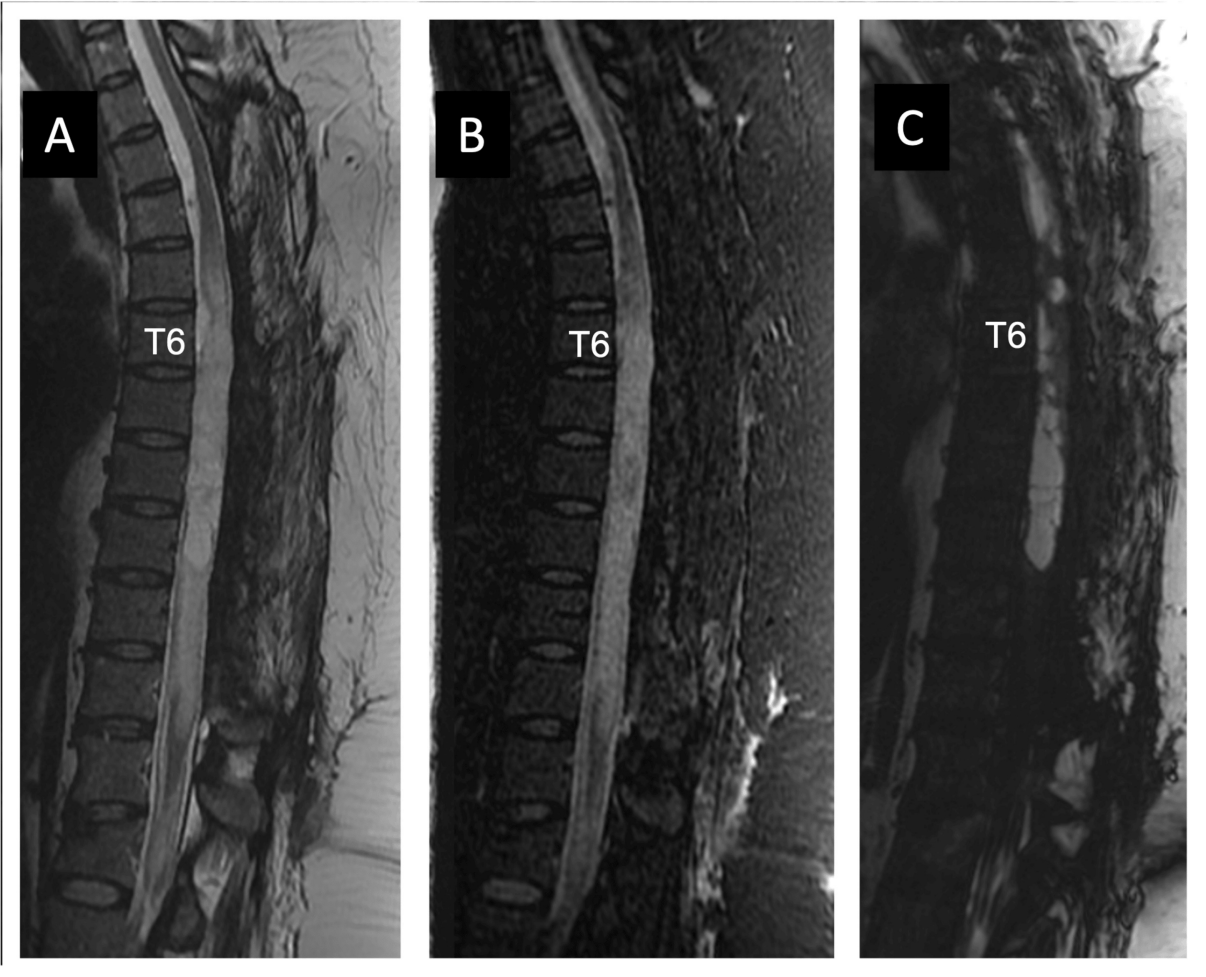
Sagittal T2 (A), T2-STIR (B), and T2-3D (C) MRI, four months after the extended laminectomy from T7 to T11 levels, shows signs of arachnoiditis with adhesions, septations within the dural sac, distortion and displacement of the thoracic spinal cord, and areas of cerebrospinal fluid encystation.
